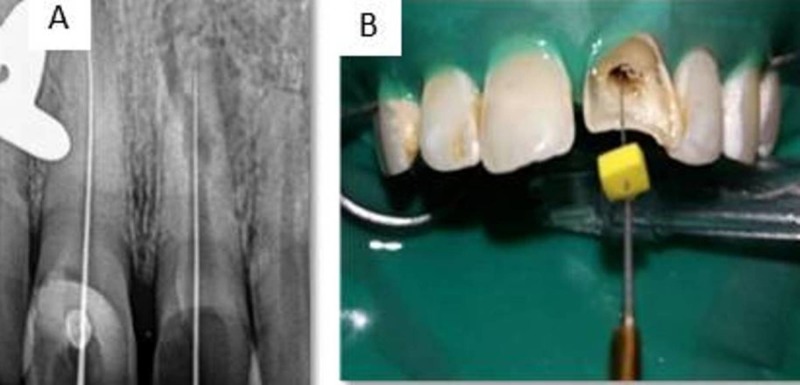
Treatment procedure on first appointment. (A) Working length radiograph for tooth 11 and 21. (B) Ultrasonic irrigation performed on tooth 21.
